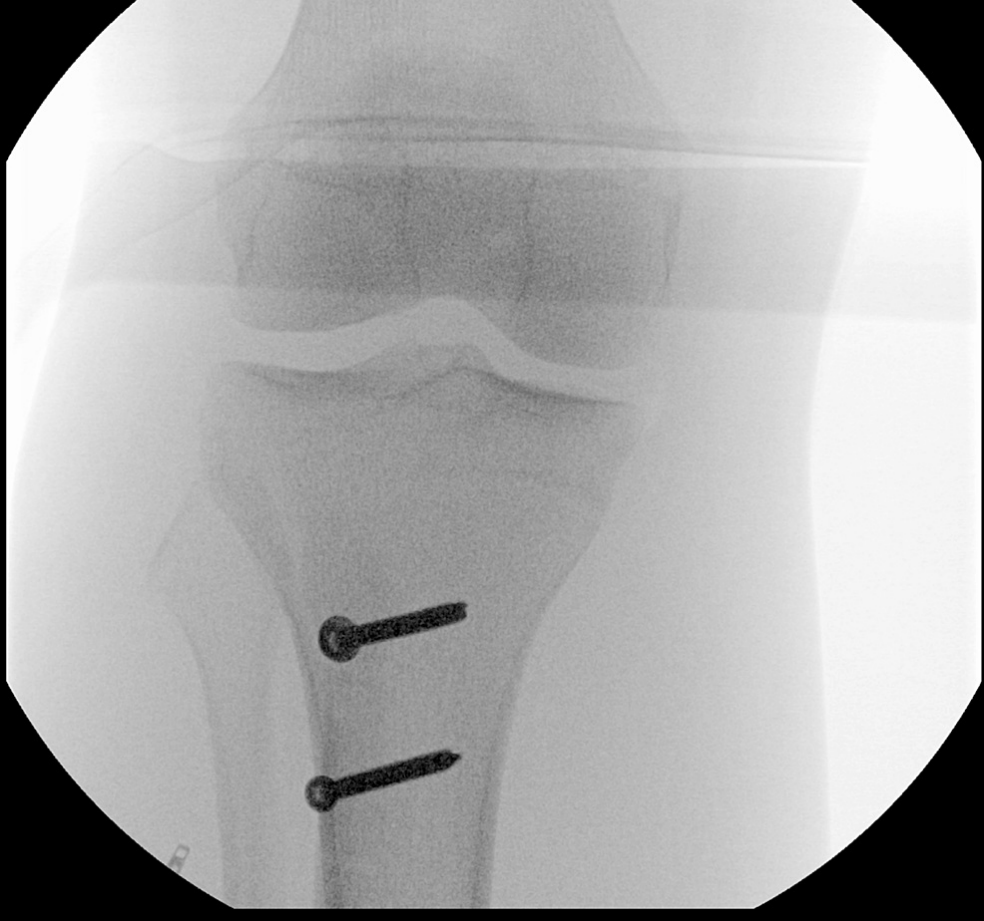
AP radiograph of the operative site at the knee. AP radiograph taken in the operating room shows no displacement of the fixation or breakage of hardware. AP, anteroposterior
